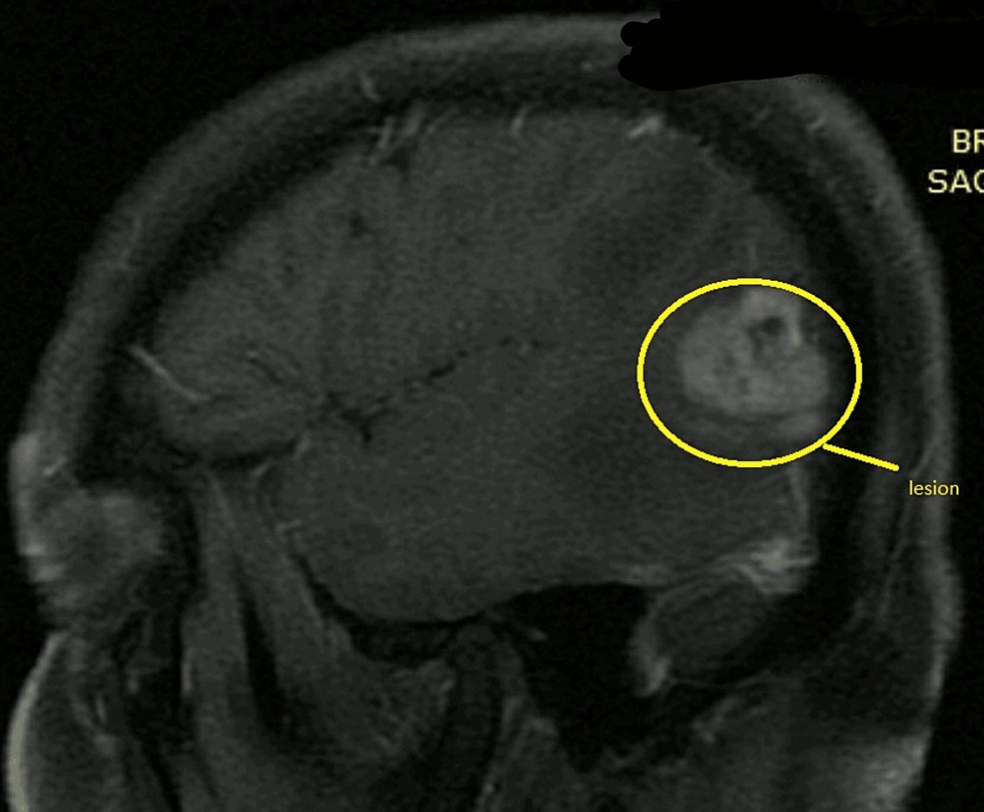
Sagittal view of the MRI showing lesion in the left temporoparietal region
